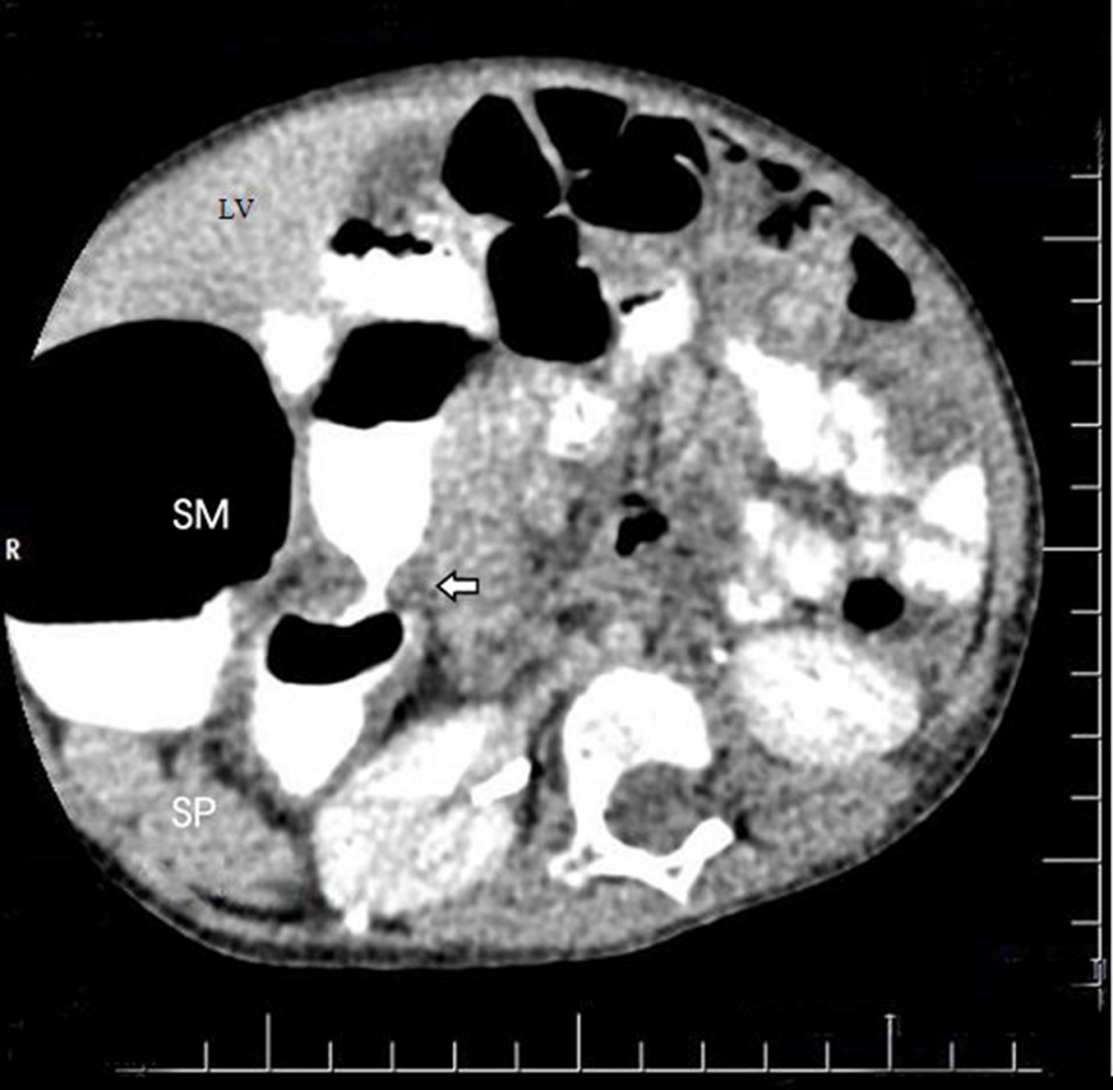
Contrast phase CT abdomen scan showing SM, LV and SP on the right. Arrow shows partial pyloric stenosis. SM, stomach; SP, spleen; LV, liver.
